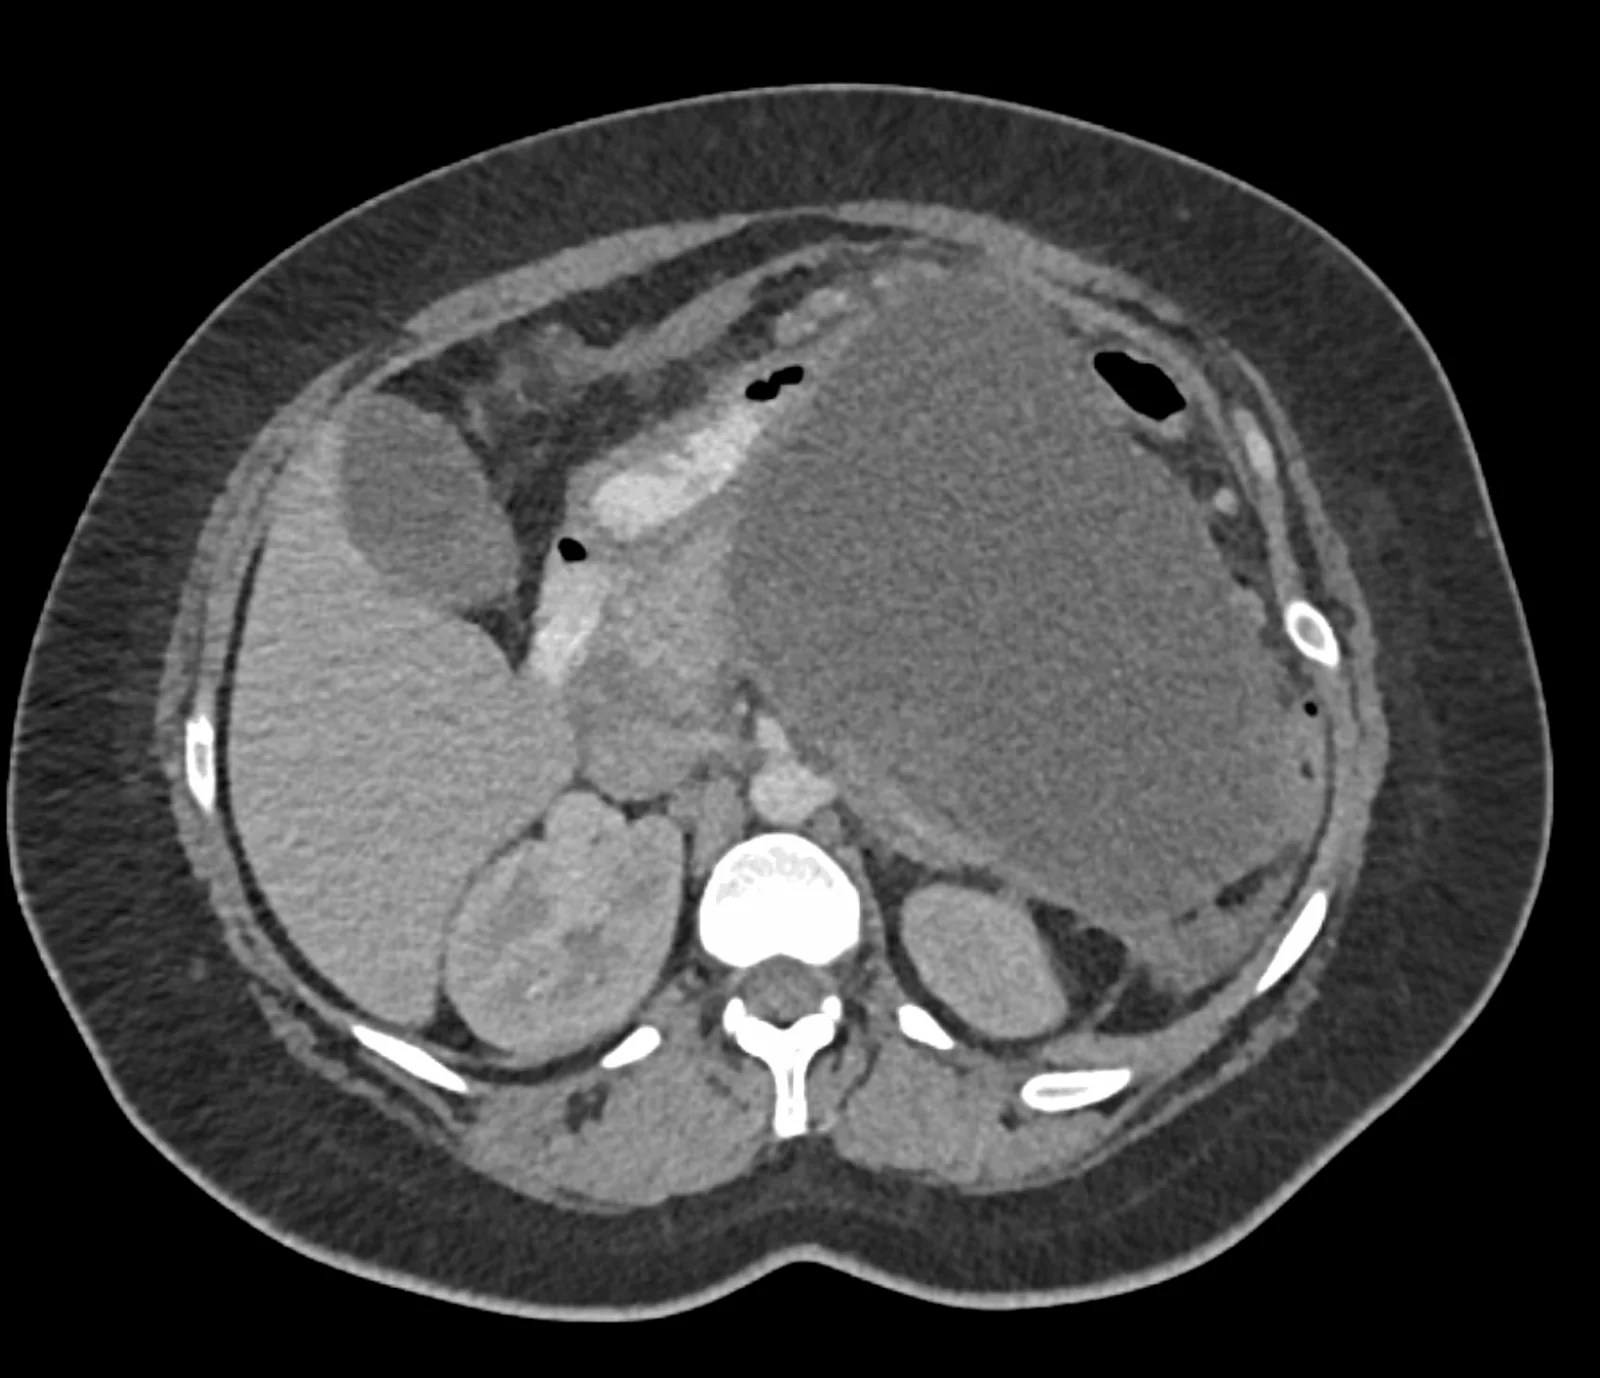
Non-contrast abdominal CT scan obtained four months after discharge. Abdominal CT demonstrating a well-defined encapsulated pancreatic collection.
